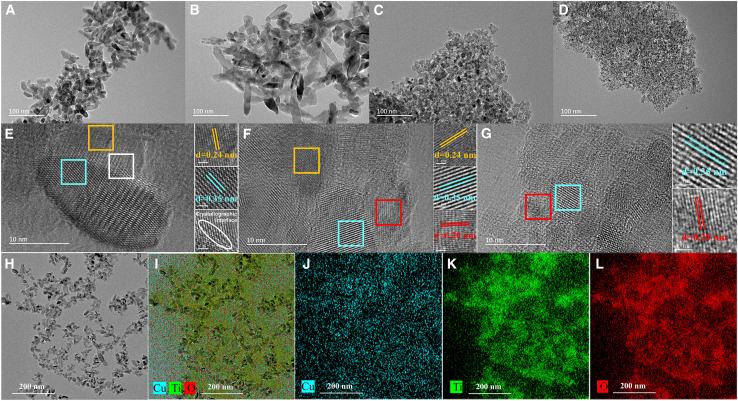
Morphology characterization (A) TEM image of CT-0.15 sample. (B) TEM image of CTC-0.5 sample. (C) TEM image of CTC-1 sample. (D) TEM image of TC-2 sample. (E) HRTEM image of CT-0.15 sample. (F) HRTEM image of CTC-1 sample. (G) HRTEM image of TC-2 sample. (H–L) EDX mapping of CT-0.15 sample.
